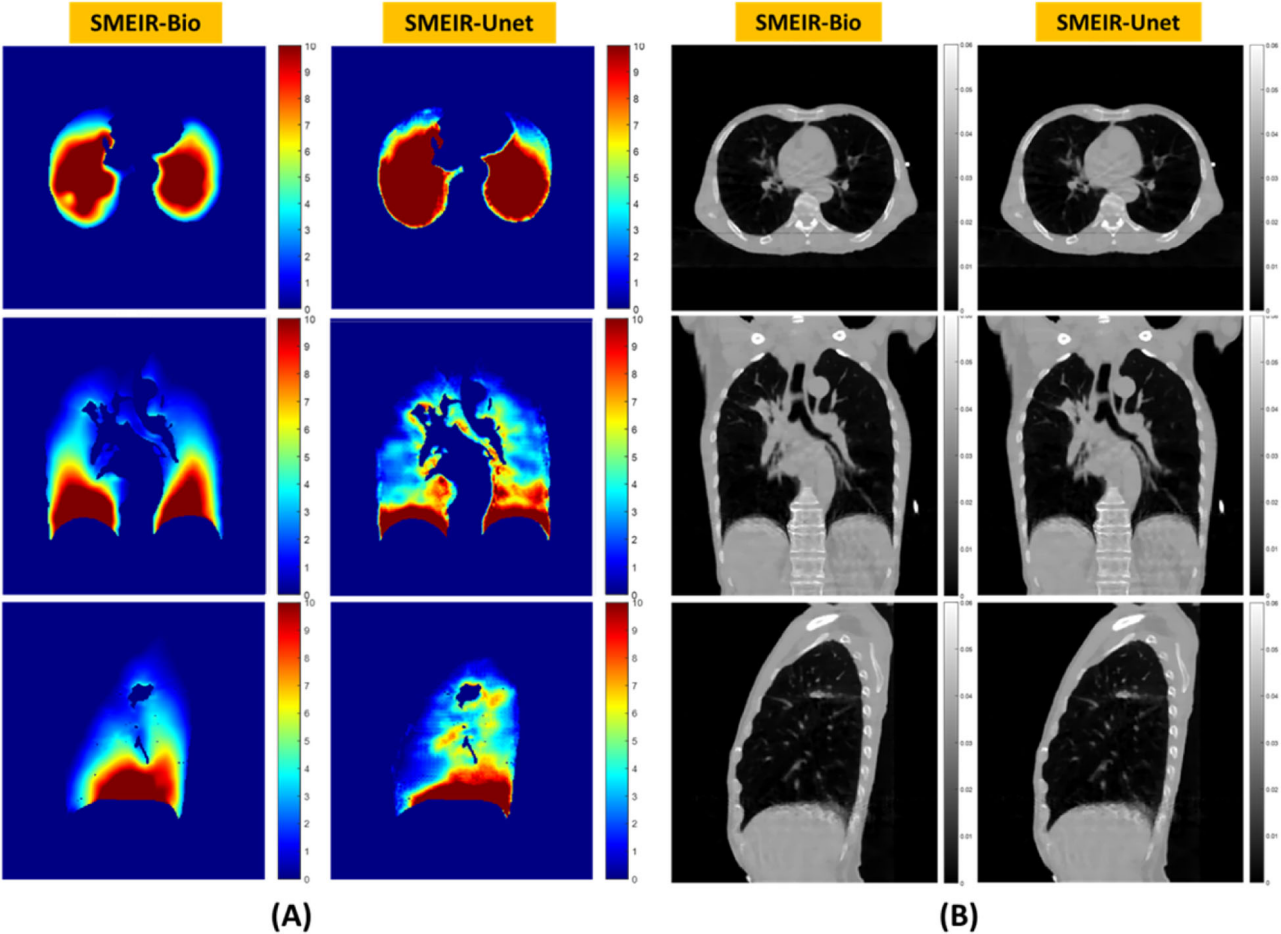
Comparison of DVFs solved by the SMEIR-Bio and the SMEIR-Unet techniques (a); Comparison of the corresponding motion-compensated CBCT images reconstructed by the SMEIR-Bio and the SMEIR-Unet techniques (b). The DVFs were from the same patient case as Fig. 6, and shown at the same slice locations. SMEIR: Simultaneous motion estimation and image reconstruction; CBCT: Cone-beam computed tomography; SMEIR-Bio: Biomechanical modeling-guided SMEIR; SMEIR-Unet: SMEIR with U-net based DVF fine-tuning; DVF: Deformation-vector-field
